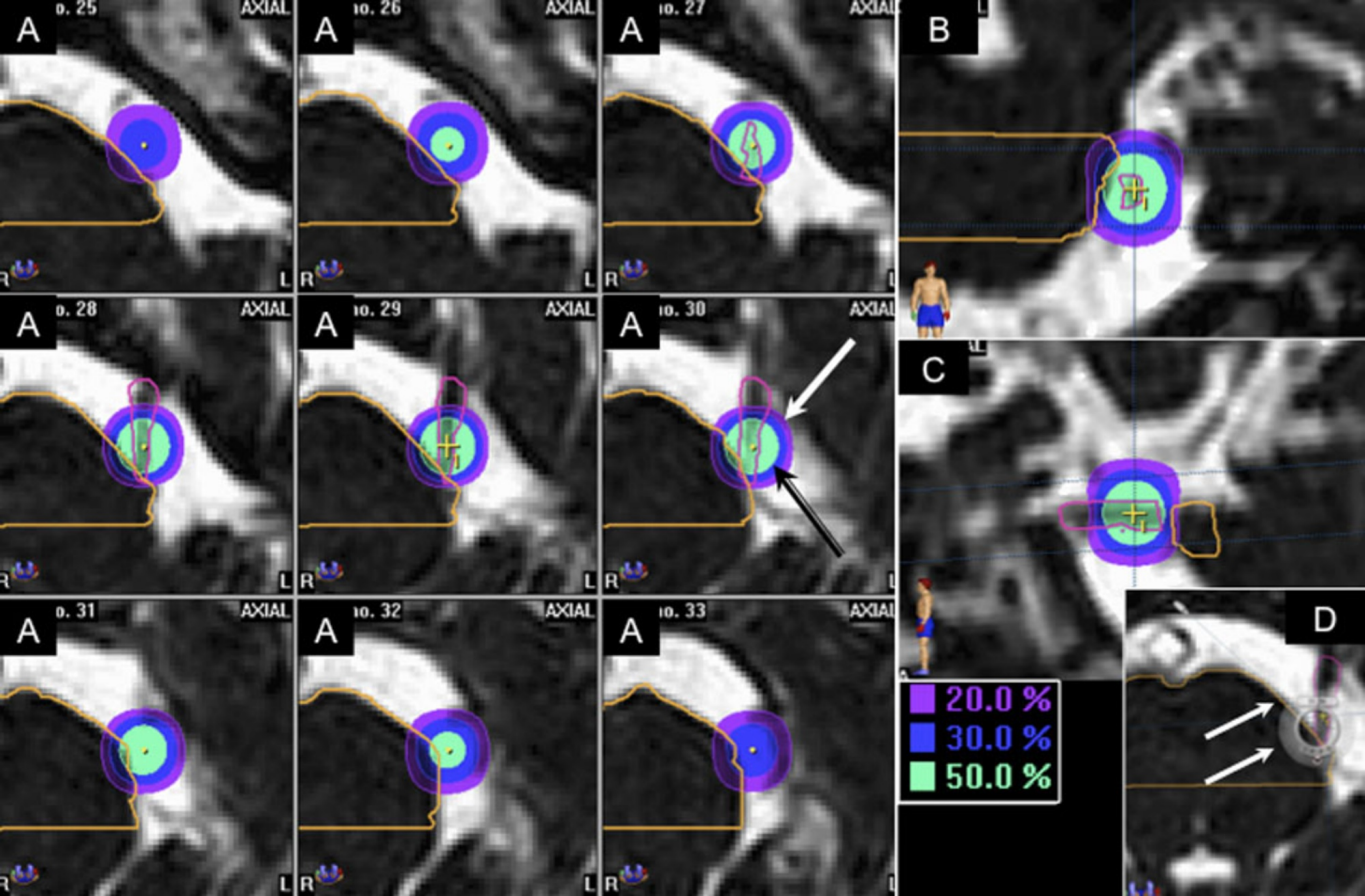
Dose distribution of the 20%, 30%, and 50% isodose lines (IDL) on the radiosurgery plan Definition of brainstem and trigeminal nerve volumes used for calculation of the dose volume histograms showing the dose distribution of the 20%, 30%, and 50% isodose lines (IDL) on the radiosurgery plan. A: axial CISS MRI sequence showing contours on nine slices. The black arrow points to the dose distribution of the 50% IDL while the white one points to the 20% IDL distribution. B and C: coronal and sagittal CISS MRI sequences of the brainstem and trigeminal nerve contours. D: The white arrows point to the digital compass positioned at the convergence of the nerve and the pons, where all measurements were taken.
